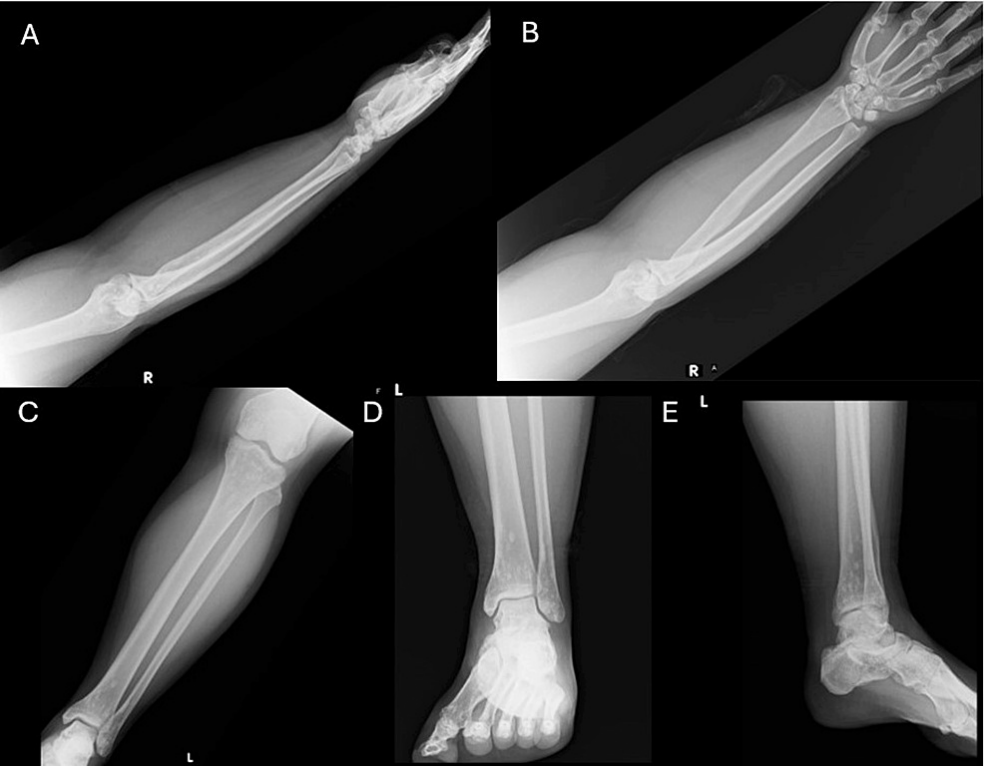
Plain radiographs of the mother, a 35-year-old woman, show several small, well-circumscribed, oval to round sclerotic lesions on the right forearm, left leg, and left ankle, which are distributed in the periarticular regions. (A) Lateral view of right forearm; (B) Anteroposterior view of right forearm; (C) Anteroposterior view of left leg; (D) Anteroposterior view of left ankle; and (E) Lateral view of left ankle.
